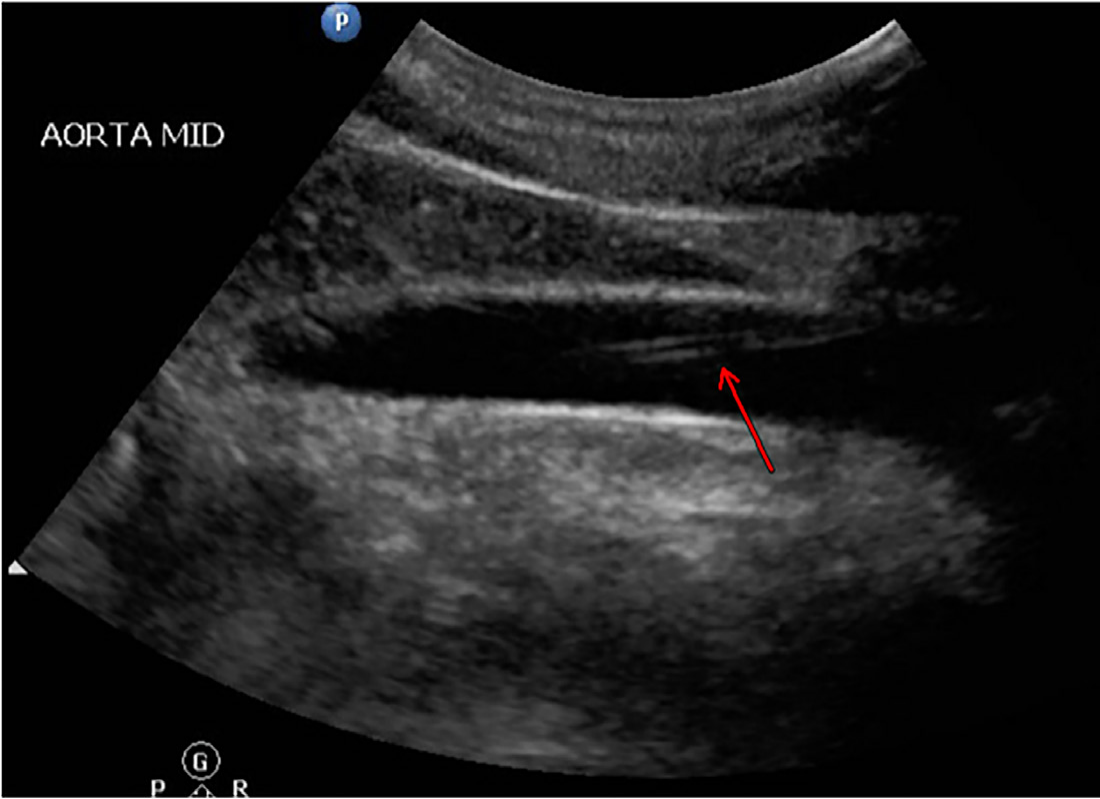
Longitudinal formal utrasonographic view of a 3.9 centimeters (cm), echogenic tubular structure with the distal tip located 1.7 cm from the bifurcation (arrow).
